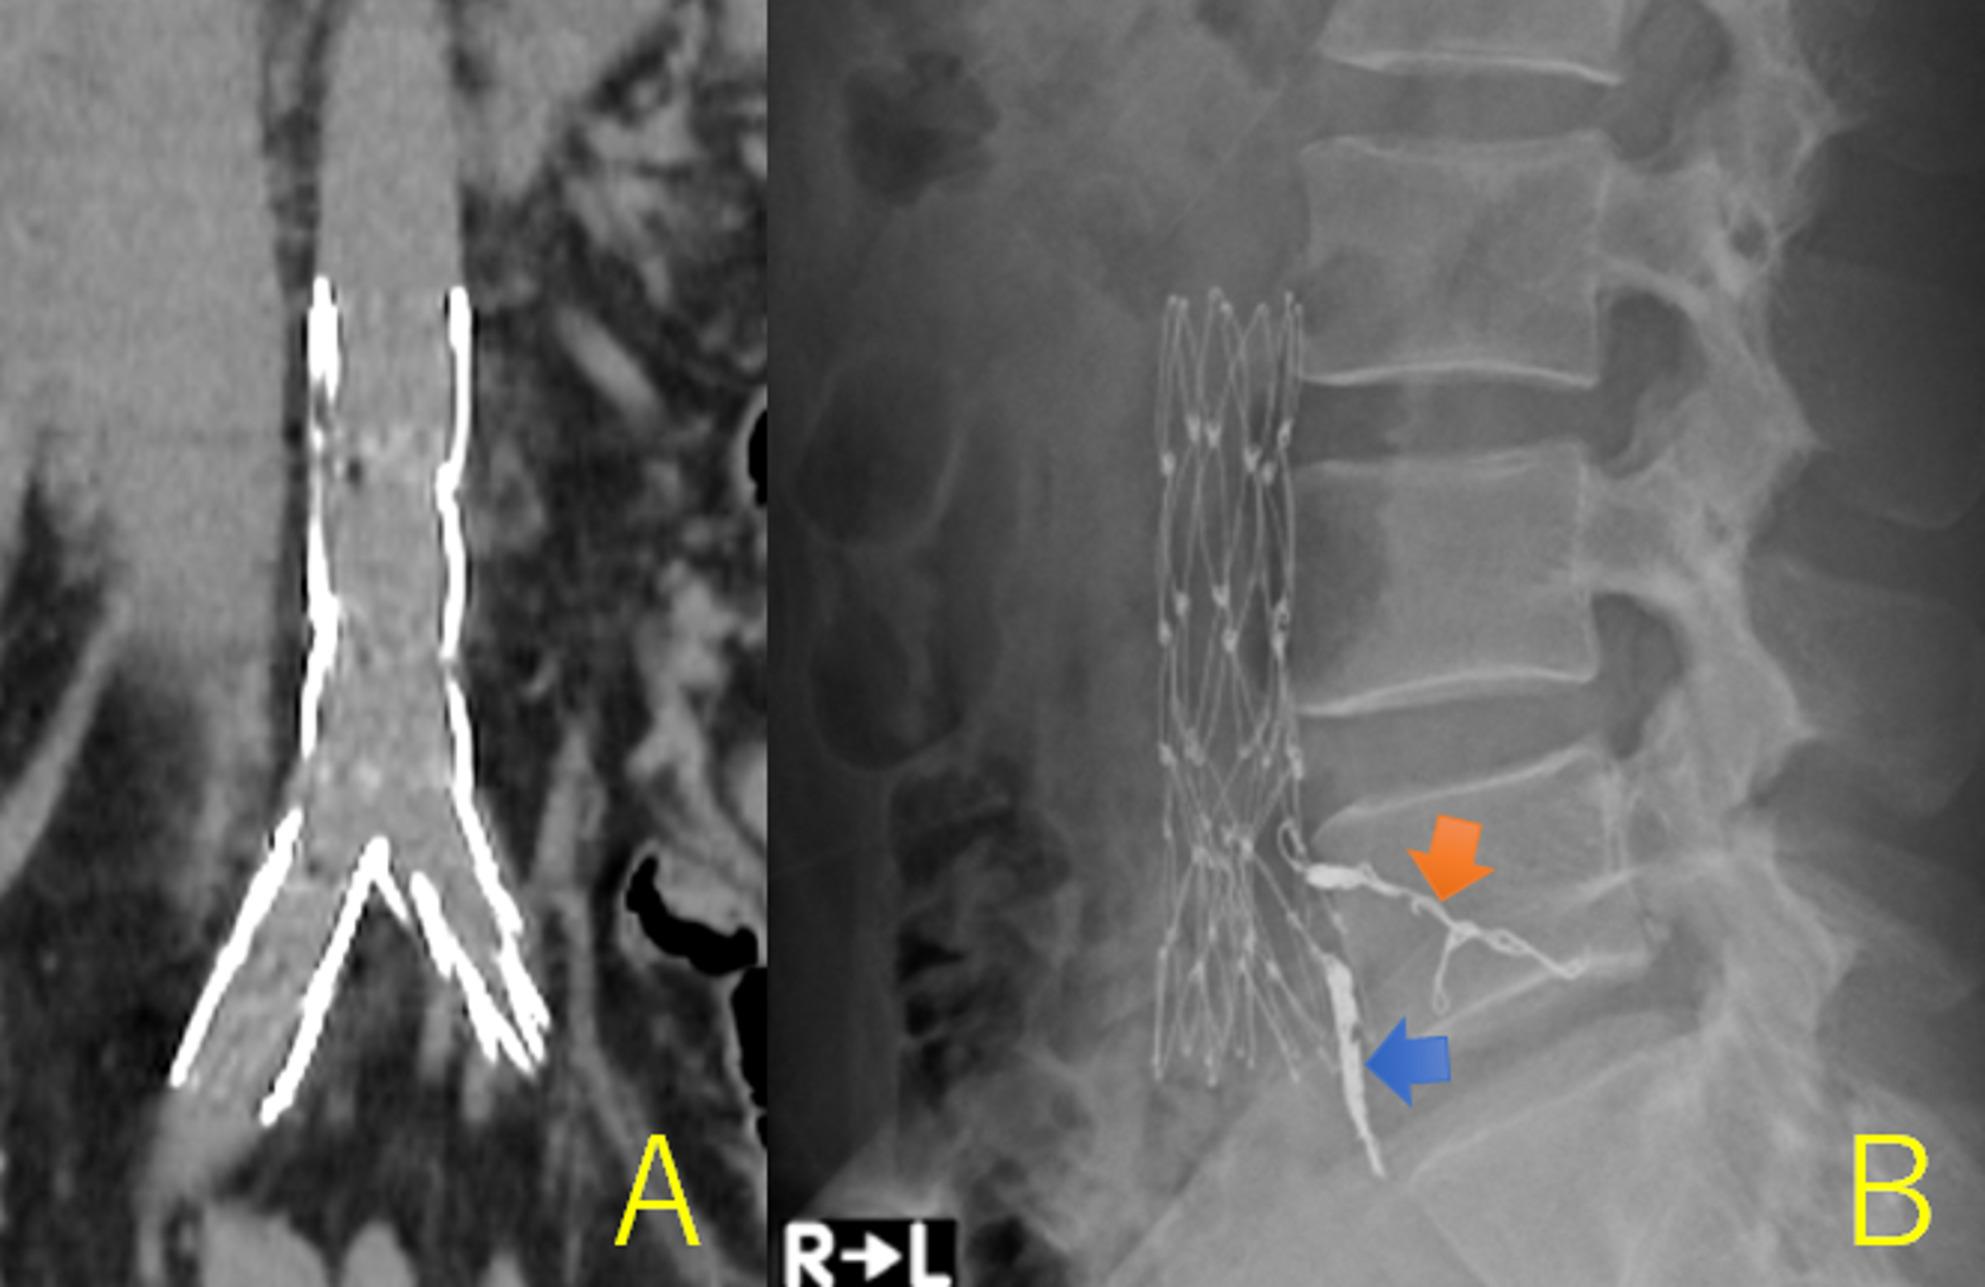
Postoperative findings. A Computed tomography showed no stent graft stenosis or migration. B A lumbar X-ray showed successful occlusion of the median sacral artery (blue arrow) and the fourth lumbar artery (orange arrow). No specific findings of ankylosing spondylitis (AS) were observed in the lumbar vertebrae or sacrum
